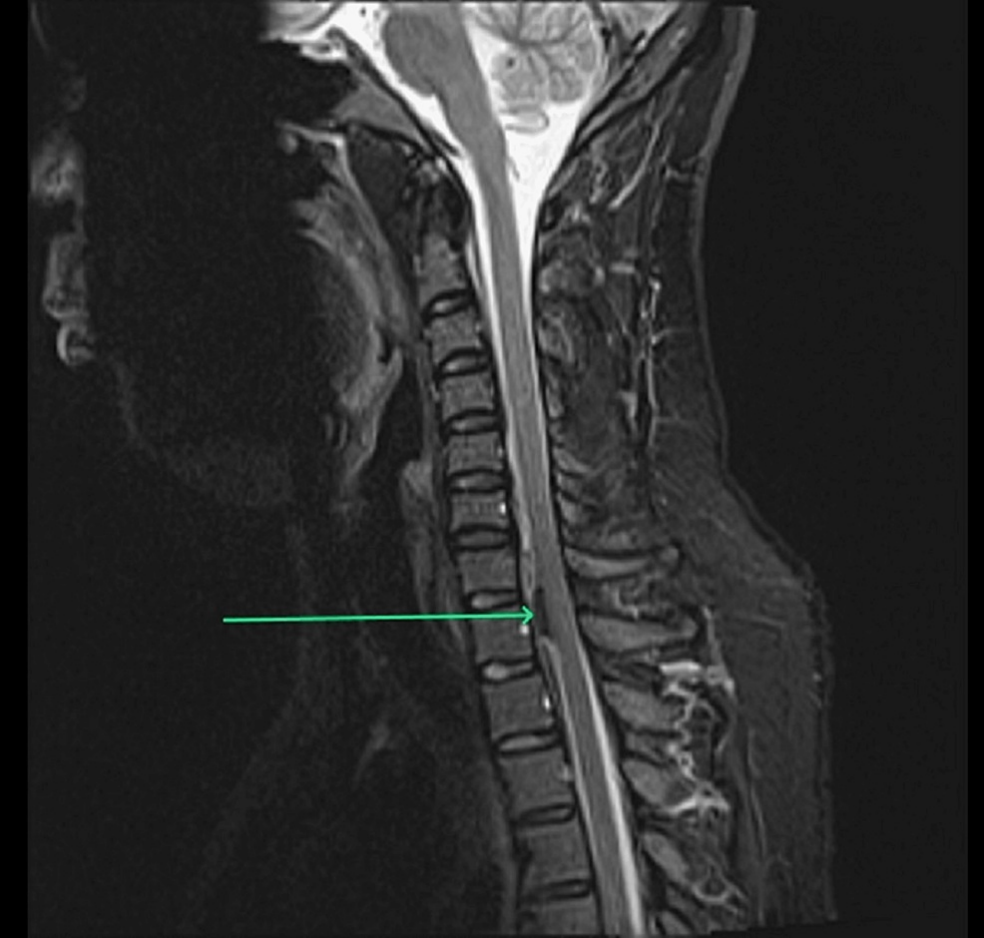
T2-weighted image of the sagittal section of the cervical spinal cord showing a well-defined extradural extramedullary hypointense lesion along the anterior surface of the spinal cord extending from mid-C7 to mid-T1 vertebral levels (arrow).
